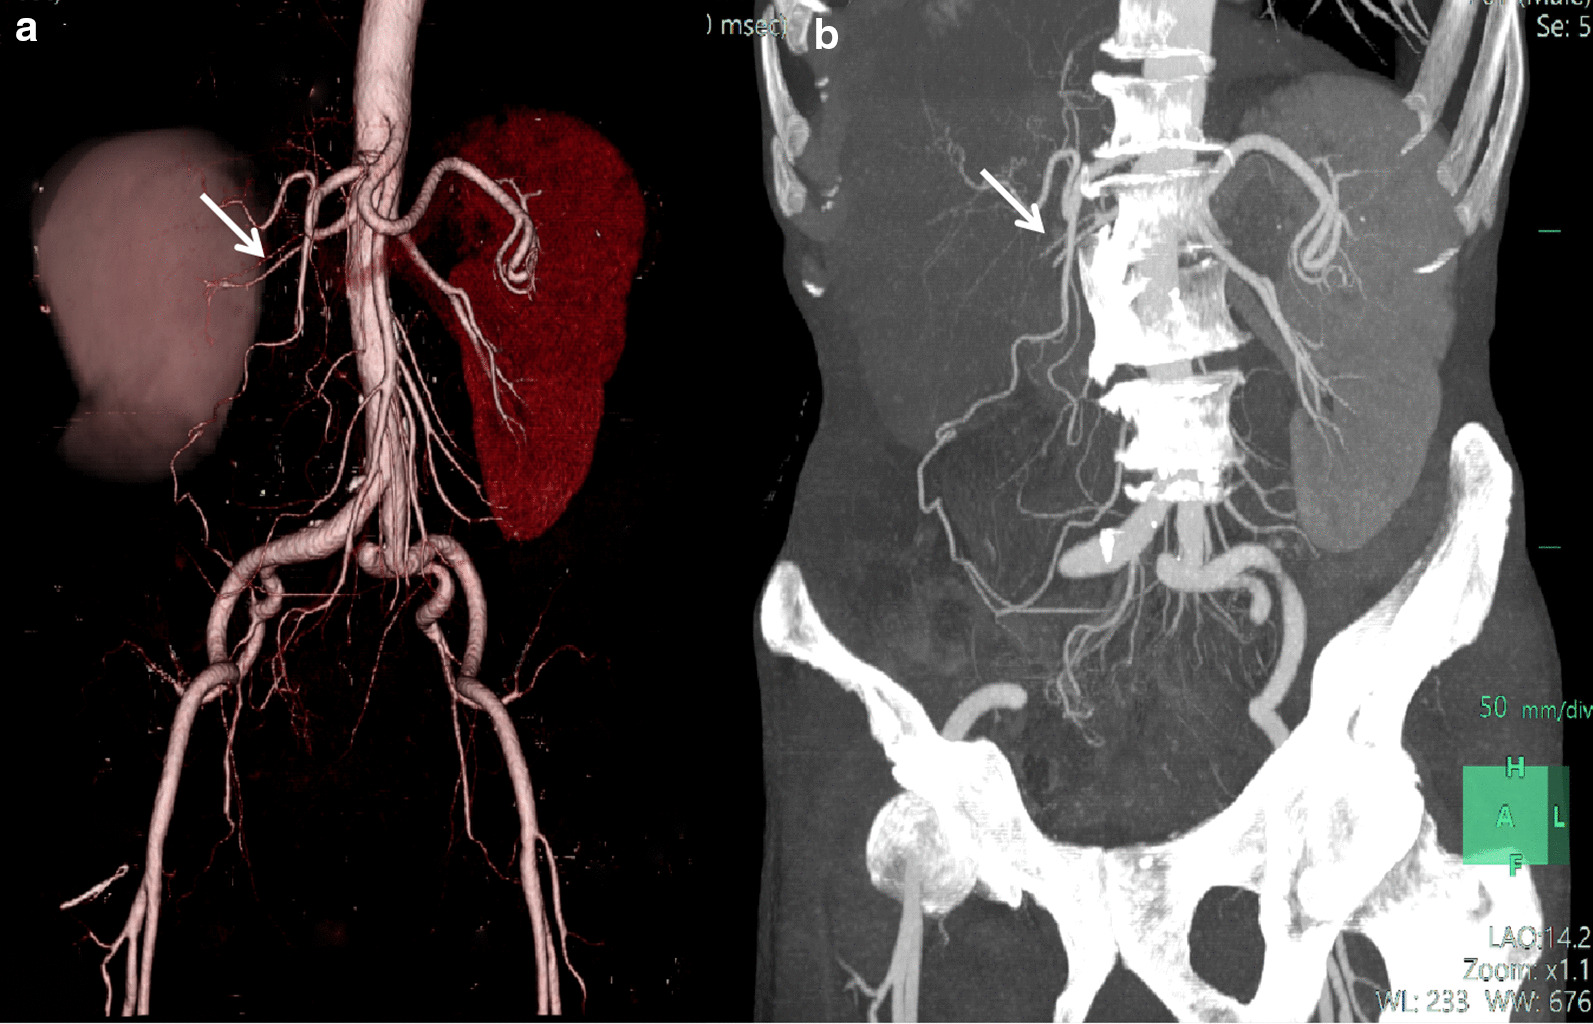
Case 5: right kidney of collecting ductal carcinoma. Volume rendering images (a) and maximum intensity projection images (b) of arterial contrast-enhanced CT show the pressure from the right renal artery (arrows)
